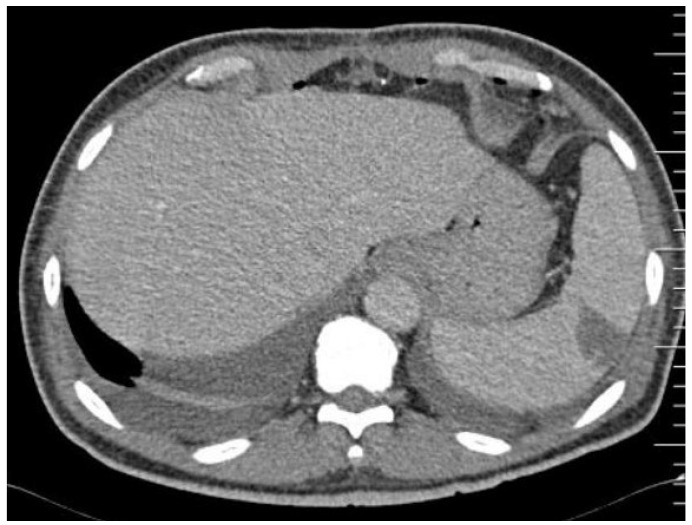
Peripheral splenic embolus on non-contrasted CT scan, wedge-shaped, subcapsular, from a patient with bicuspid aortic valve endocarditis caused by *Abiotrophia defectiva*. Source: Instituto Nacional de Cardiologia image collection, 2017.
